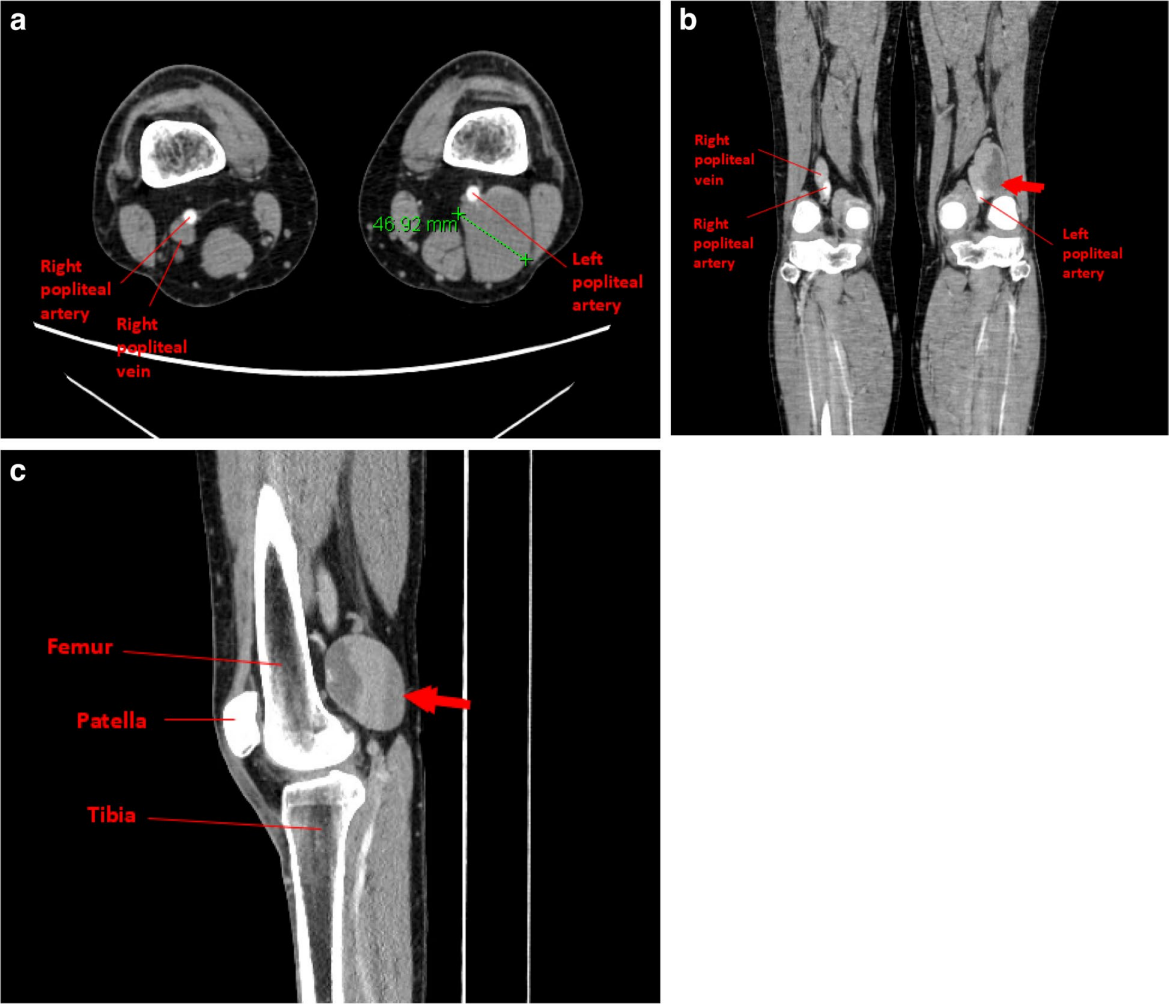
a axial CT angiogram showing left popliteal vein aneurysm measuring 46.92 mm. b coronal image showing left popliteal vein aneurysm (arrow), with mural thrombus within aneurysmal segment. c Sagittal CT image showing left popliteal vein aneurysm (arrow)
